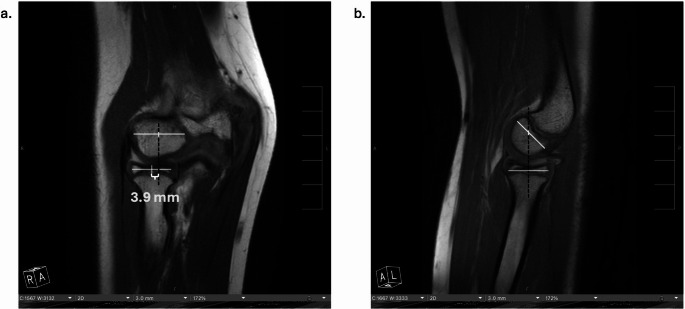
Exemplar measurements of the radiocapitellar offset in the coronal and sagittal planes. (a) Coronal MRI demonstrating radial (positive) radiocapitellar offset of 3.9 mm. The maximal medial-lateral dimension of the ossified radial head (dashed lines) and capitellum (solid lines) are shown with hash marks at the true center. (b) Sagittal MRI demonstrating no radiocapitellar offset. The maximal anterior-posterior dimension of the ossified radial head (dashed lines) and capitellum (solid lines) are shown with hash marks at the true center
